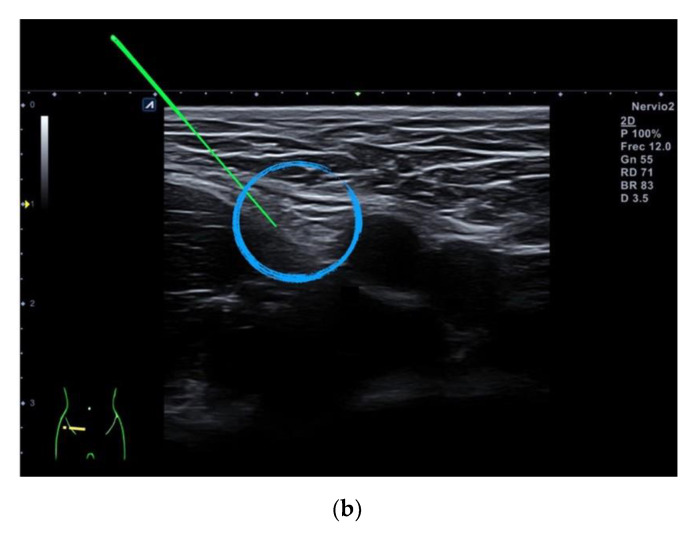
Femoral nerve ultrasound location for applying PENS over the left lower extremity: (a) Transducer placement; (b) ultrasound imaging of the femoral nerve.
